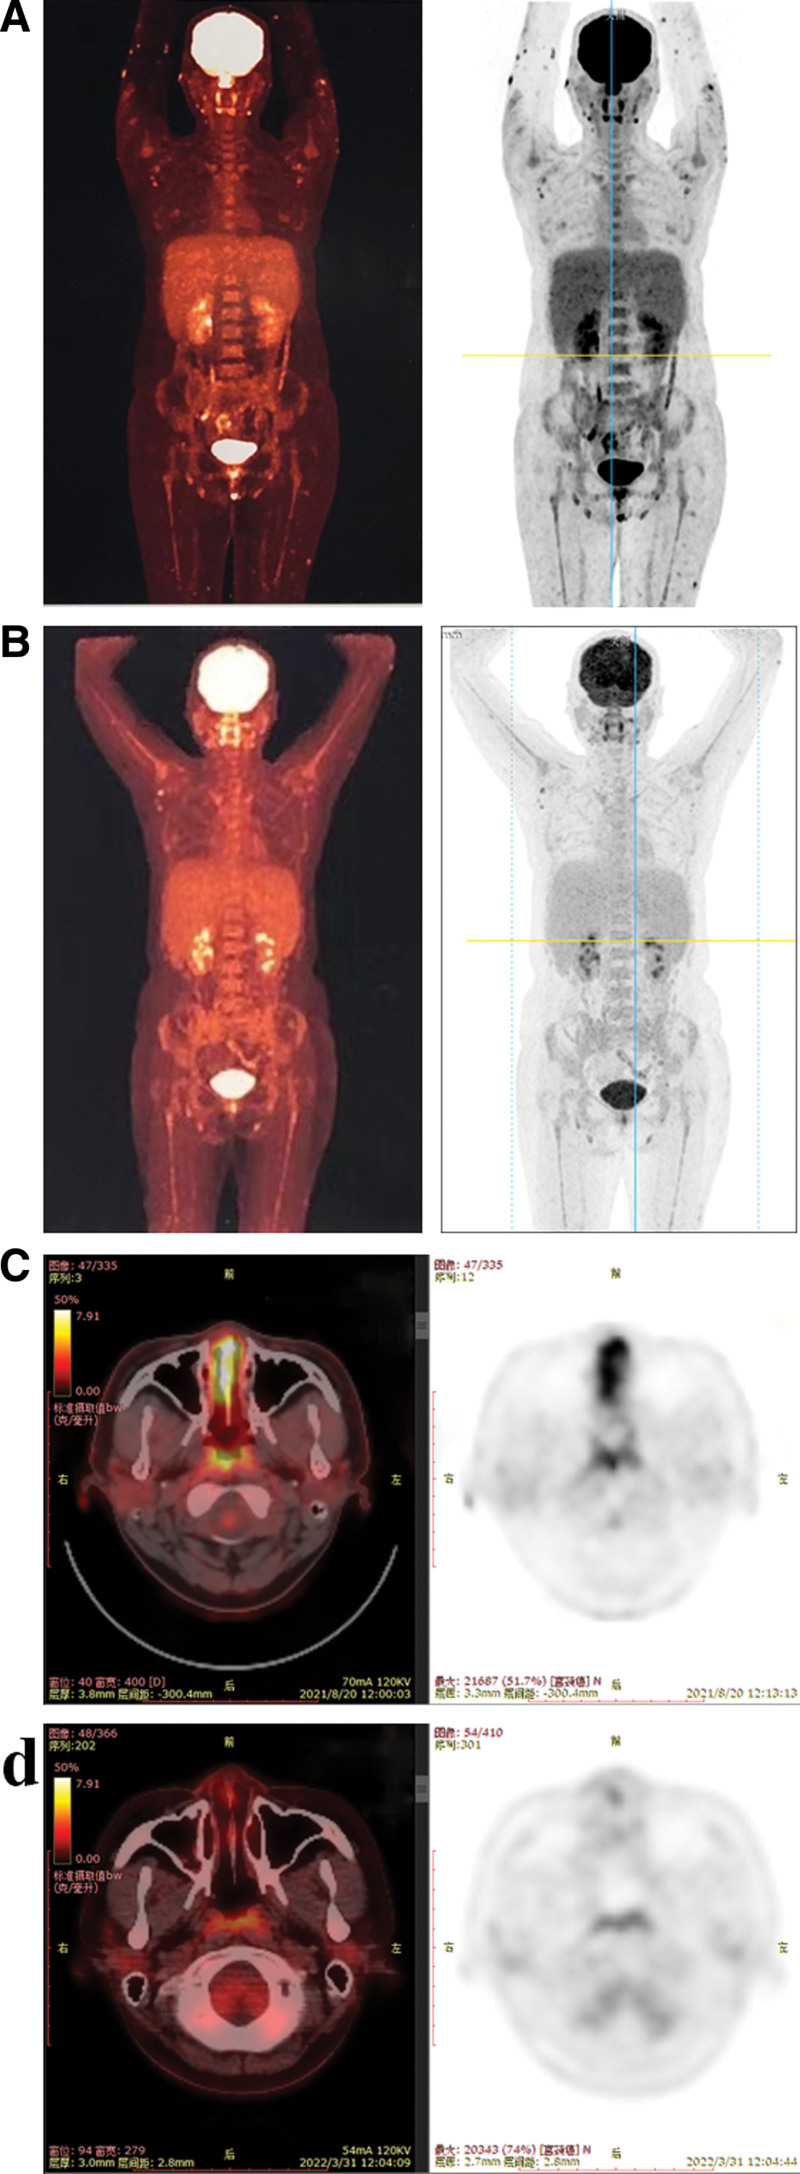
Positron emission tomography computed tomography (PET-CT) images. (A) Multiple cutaneous nodules in the whole body with increased glucose metabolism before leprosy therapy. (B) The cutaneous lesions after leprosy therapy. (C) Thickened nasal mucosa with increased glucose metabolism before leprosy therapy. (D) The lesions of nasal mucosa after leprosy therapy.
